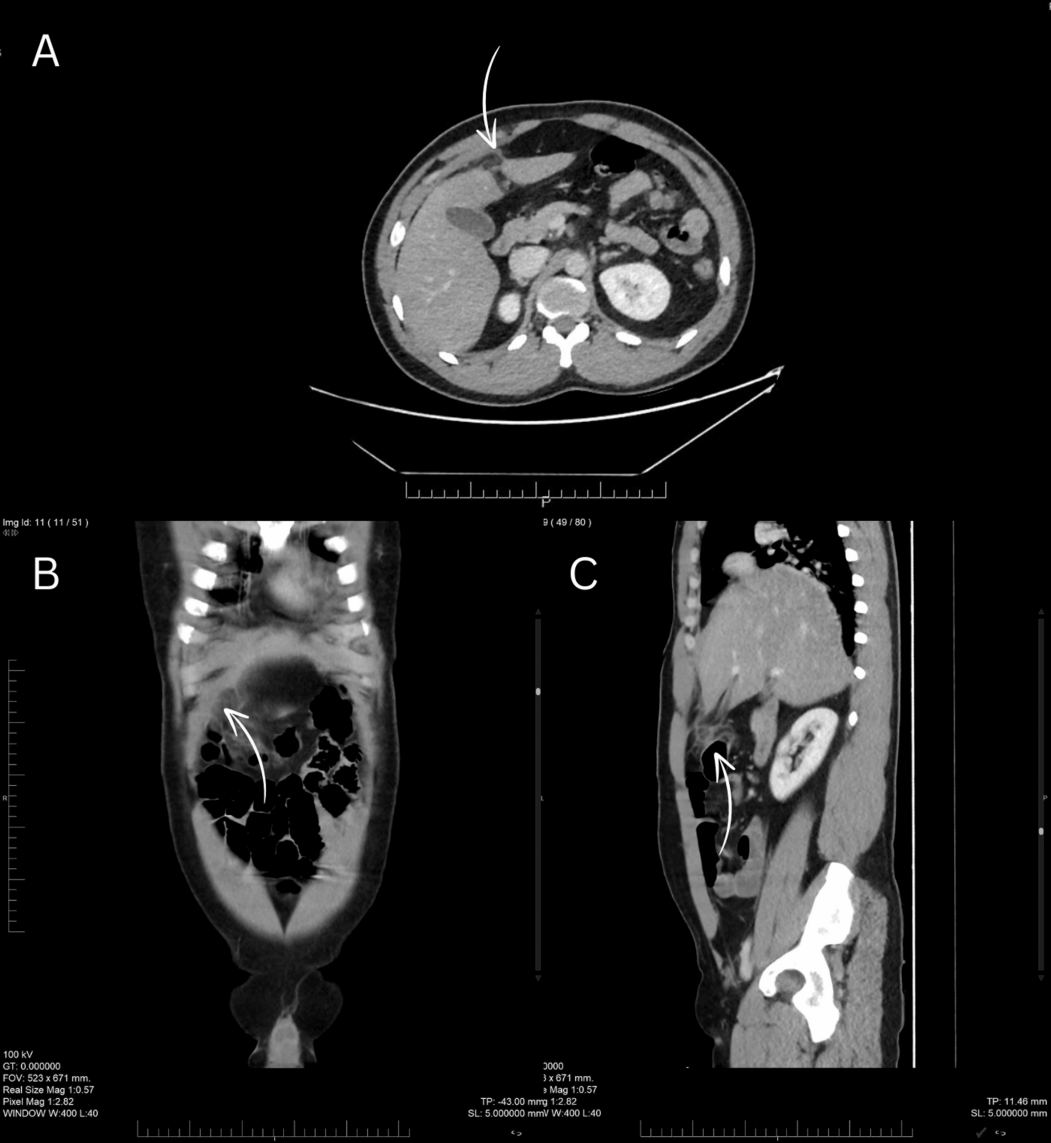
Contrast-enhanced CT scan of the abdomen showing features suggestive of falciform ligament appendagitis. (A) Axial, (B) coronal, and (C) sagittal views demonstrate a fat-attenuated oval lesion with surrounding inflammatory changes adjacent to the falciform ligament (arrows).
